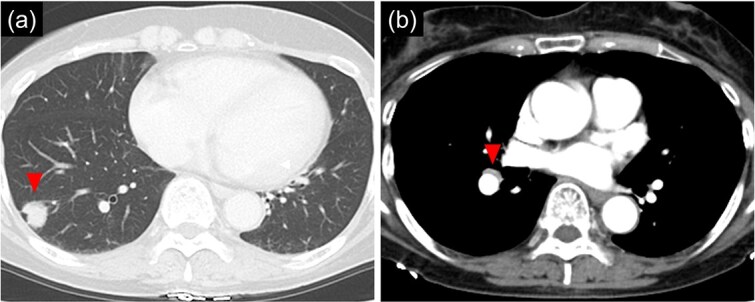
Chest computed tomographic image. (a) An 18 mm size nodule is detected in the S9 region of the right lower lobe. (b) An enlarged lymph node (#11iLN) with fluorodeoxyglucose accumulation is located anterior to the basal segment of the pulmonary artery.
